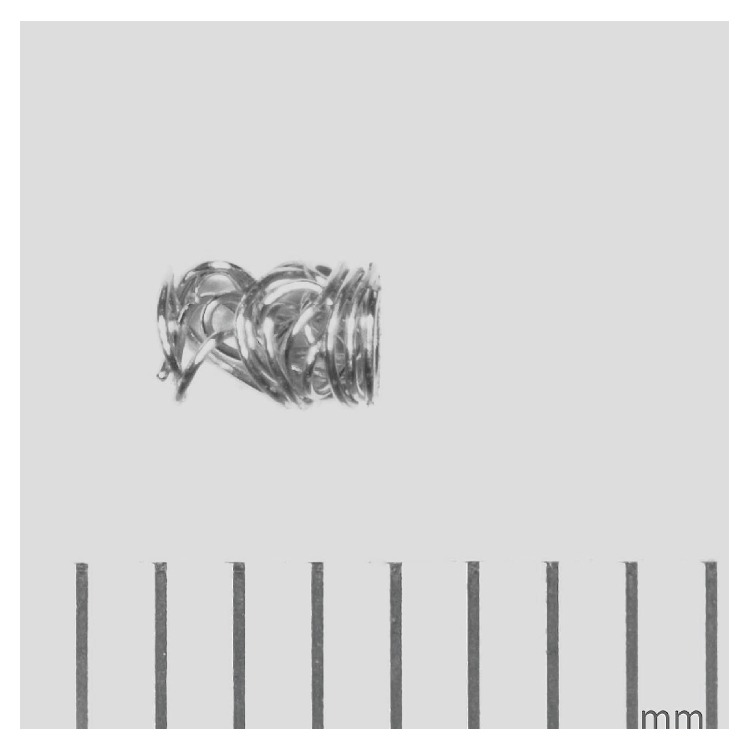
The metallic tissue marker (Achieve Marker, 3-mm length, CareFusion, Vernon Hills, IL) that underwent testing for MRI issues at 3 Tesla. Note the irregular surface, allowing for growth of tissue in and around this implant to reduce migration once it is deployed.
